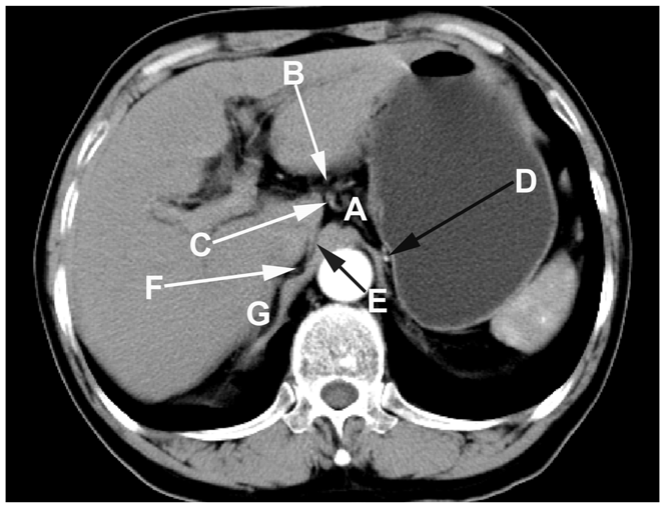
Manifestation of the LES on axial CT image from the inferior. A: LES; B: lesser omentum; C: left gastric artery; D: posterior gastric artery; E: narrow space; F: hepatic bare area; G: right adrenal gland.
